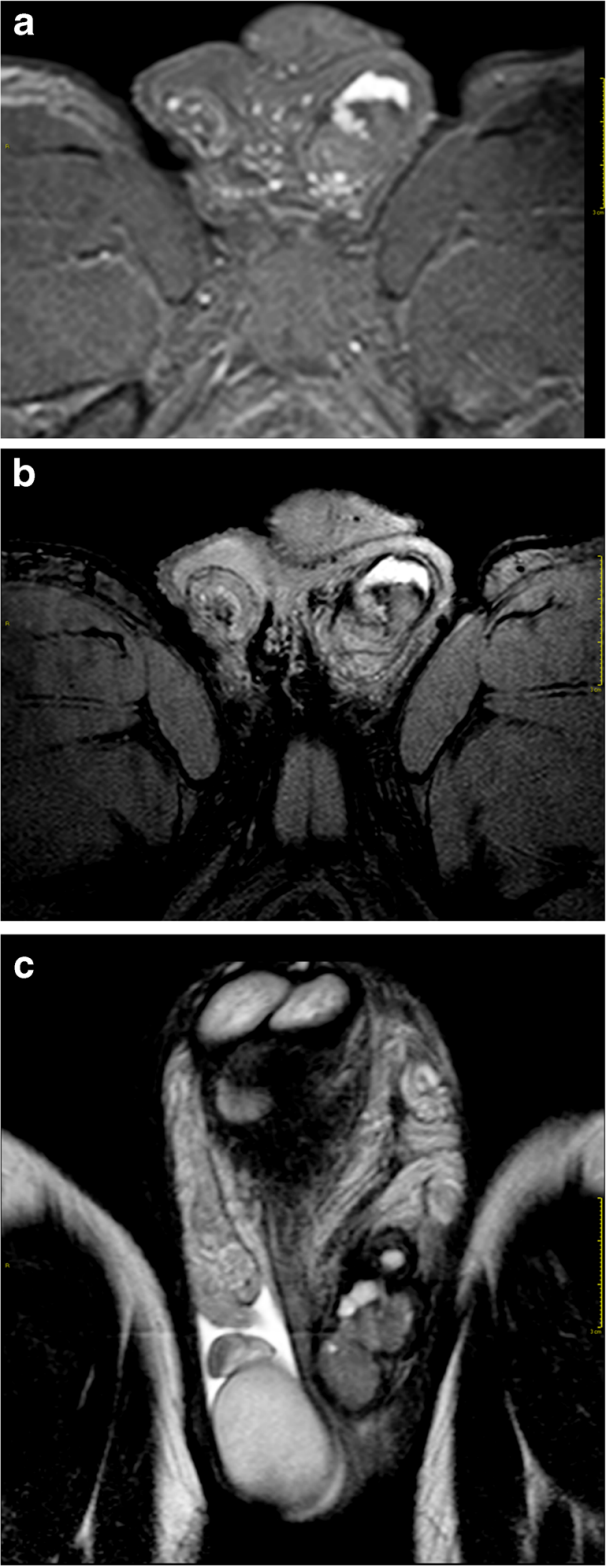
a-c Left testicle rupture. MRI shows a tunica albuginea tear and testicle rupture. a T1-weighted SPIR TSE sequence, axial view, b T2-weighted FFE sequence, axial view; c T2-weighted TSE sequence, coronal view
